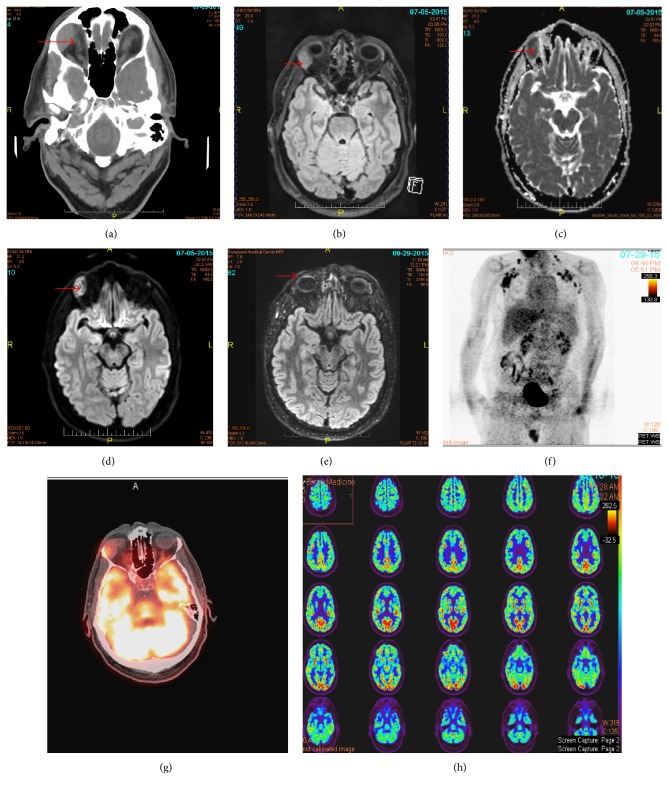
Head CT revealing a lacrimal gland mass (a). Lacrimal gland mass on initial Axial FLAIR (b). Restricted diffusion in the lacrimal glass mass (c and d). Repeat MRI after initiation of prednisone revealing decreased size of lacrimal lesion (e). Hypermetabolic foci corresponding to lymph nodes in bilateral neck, axillary regions, chest, pelvis, and inguinal regions on whole-body PET/CT (f). Prominent hypermetabolic lesion in the right lateral orbit before prednisone (g). No evidence of hypermetabolic lesions or regional hypometabolism within the brain on PET/MRI 5 months after prednisone and infliximab (h).
